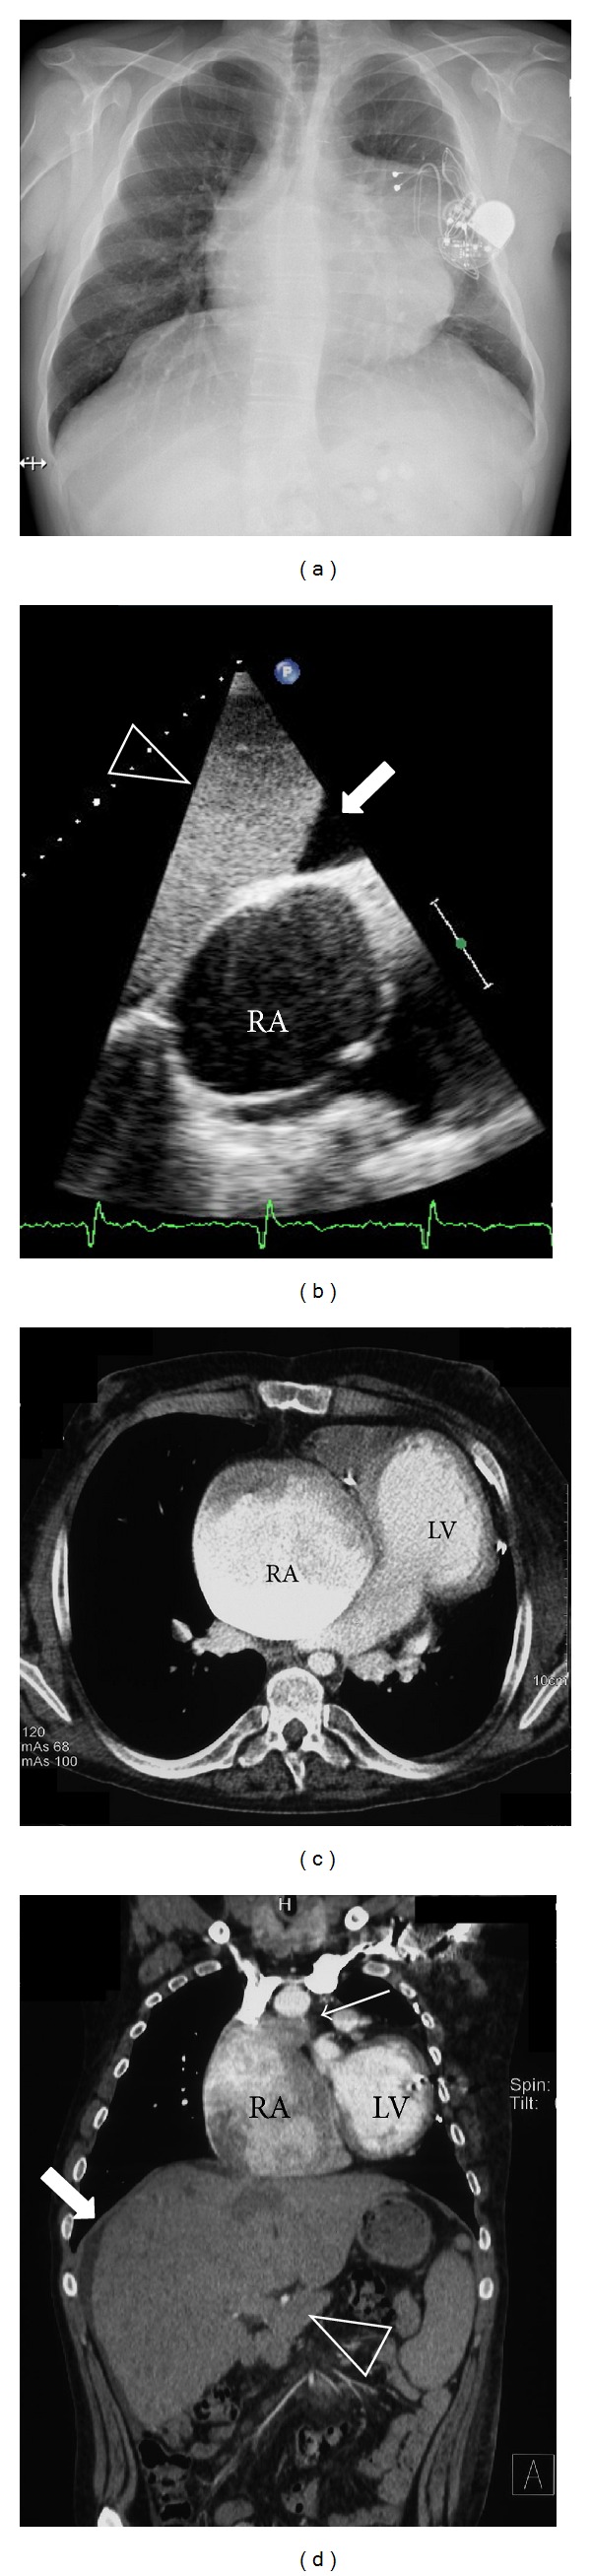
31-year old patient with tricuspid atresia, atrial and ventricular septal defects, and severe infundibular pulmonary stenosis. He underwent surgical palliation with a Fontan procedure, initially using an atrial to right ventricular conduit, later converted to an atriopulmonary Fontan-Kreutzer-Anastomosis due to conduit failure. After increasing heart failure and 5 cardiac surgeries, he underwent heart transplantation. (a) Chest X-ray with massive cardiac enlargement and the epicardial pacemaker. (b) Subcostal echocardiographic view showing severely dilated right atrium (RA) with spontaneous echocontrast and perihepatic ascites (bold arrow). (c) Axial plane of chest-computed tomogram showing giant right atrium (RA) and normal-sized left ventricle (LV). (d) Coronal plane of chest and abdominal computed tomography showing enlarged right atrium (RA) with connection to the main pulmonary artery (arrow) and hepatomegaly (arrow head).
